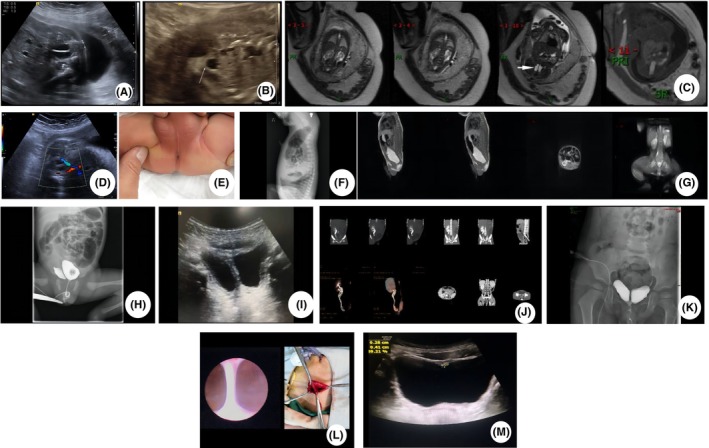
(A, B) Prenatal ultrasound at 27.5 weeks gestation showing abnormal septum in bladder and left multicystic kidney. (C) Prenatal MRI showing duplicated bladder. (D) Repeat prenatal ultrasound at 31 weeks gestation showing abnormal septum in bladder. (E) Physical examination at birth showing anal atresia. (F, G) The inversion radiography and MRI on Day 1 of the life showing anal atresia. (H) Voiding cystourethrography (VCUG) showing a filling defect separating the bladder into two halves. (I) A repeat ultrasound at 1 year old clearly showing abnormal septum. (J) CT urography at 3 years old showing a left renal hypoplasia, a right duplex kidney and incomplete bladder duplication. (K) VCUG at 3 years old showing incomplete bladder duplication. (L) The septum which separate the two bladders was confirmed during the surgery. (M) Ultrasound revealed regular bladder morphology.
